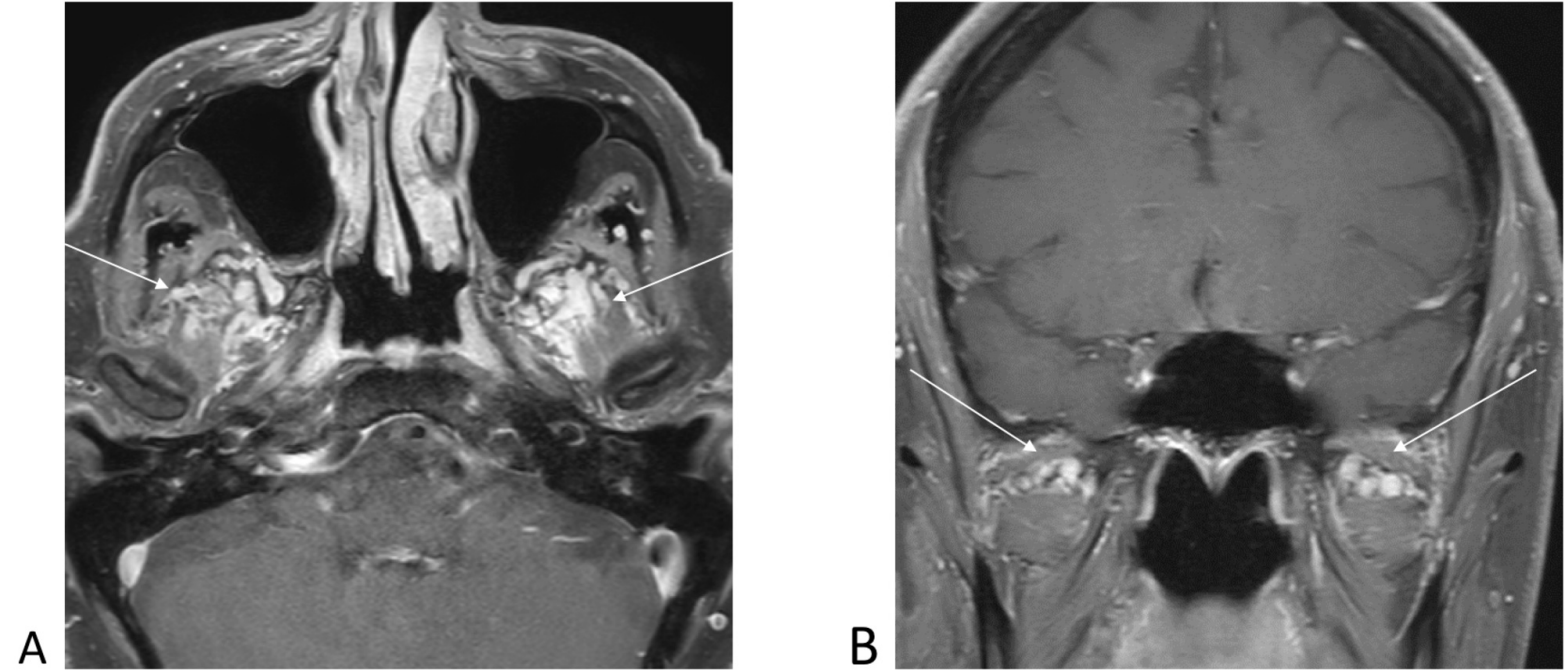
Post-contrast T1-weighted magnetic resonance images demonstrating prominent pterygoid plexus varices noted bilaterally (arrows). 2A, axial T1-weighted with contrast. 2B, coronal T1-weighted with contrast.
